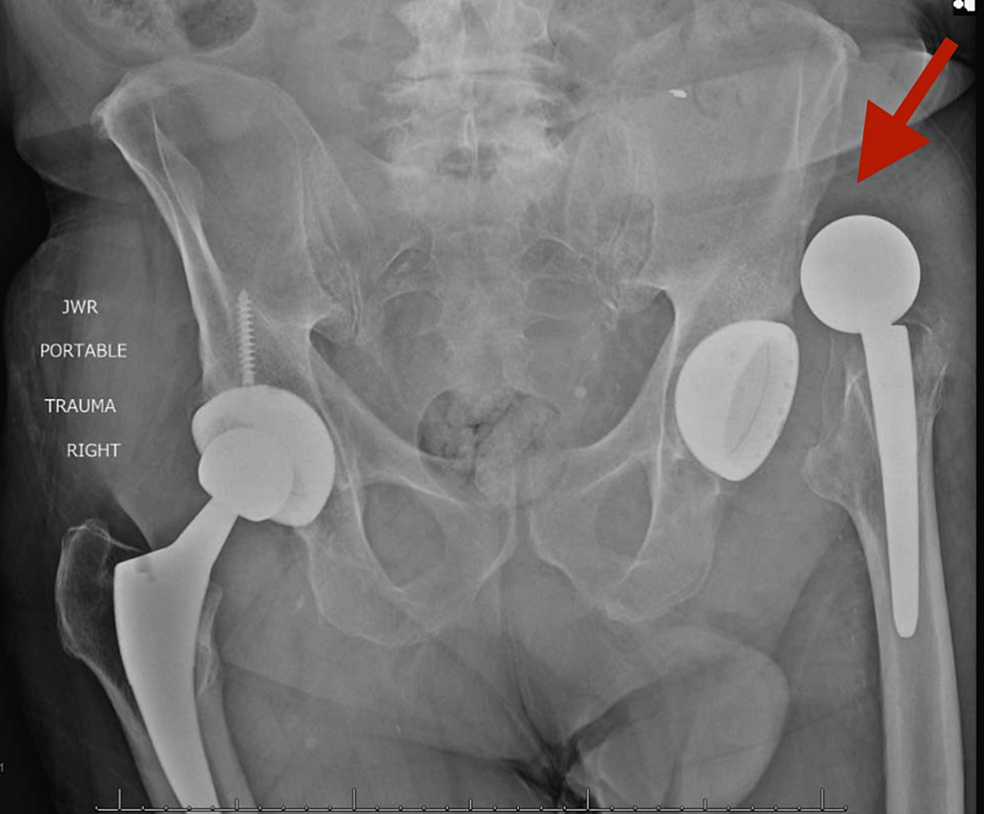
AP pelvis radiograph demonstrating superior dislocation of left THA AP, anterior-posterior; THA, total hip arthroplasty
